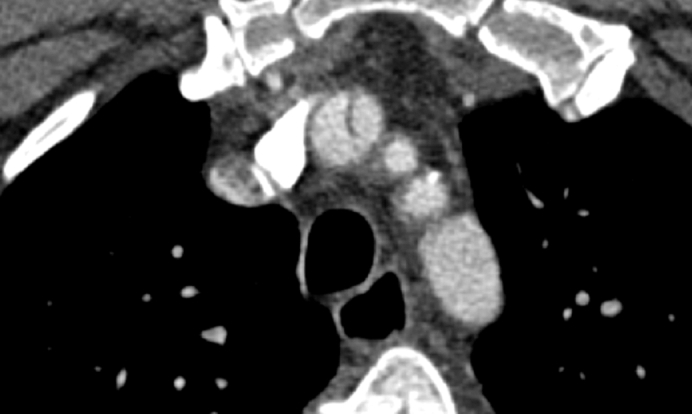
Innominate artery false lumen communication as demonstrated on preoperative CT.
